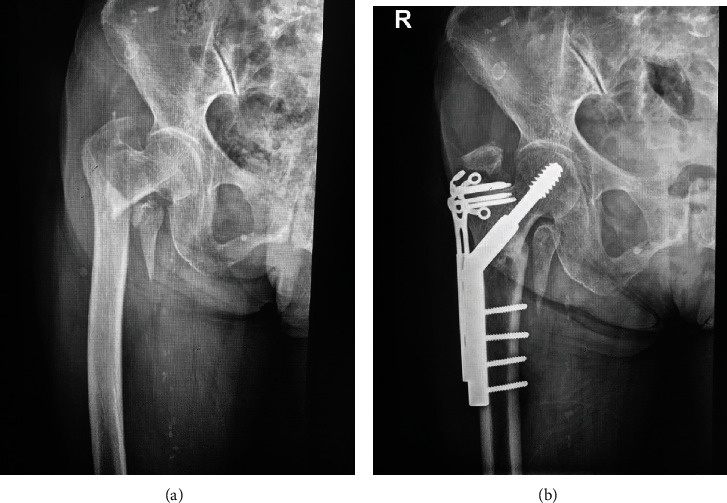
Preoperative and postoperative anteroposterior plain radiographs of a 31A2 fracture treated with DHS+TSP. (a) Preoperative and (b) postoperative anteroposterior plain radiographs of a 31A2 fracture treated with dynamic hip screw + trochanter-stabilizing plate. (b) Postoperative radiographs showing osseous union over the fracture site but greater trochanteric tip avulsion fractures.
